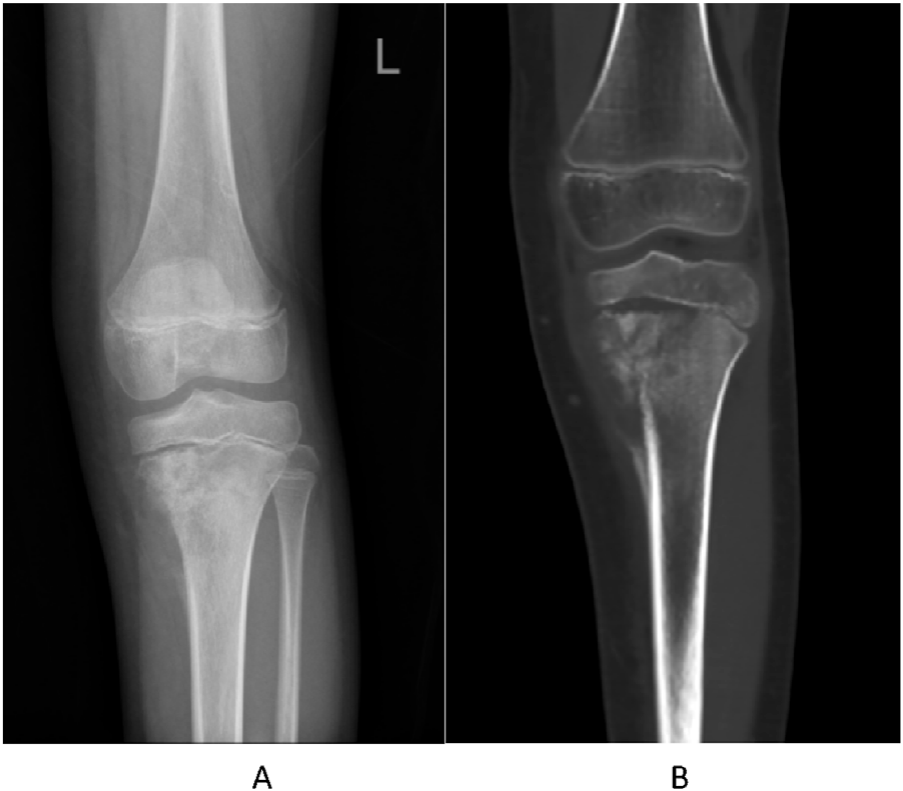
Pre-treatment imaging of the left proximal tibia. (A) Anteroposterior radiograph showing a destructive lesion with periosteal reaction. (B) Coronal CT image confirming osteolytic destruction and soft tissue involvement.
